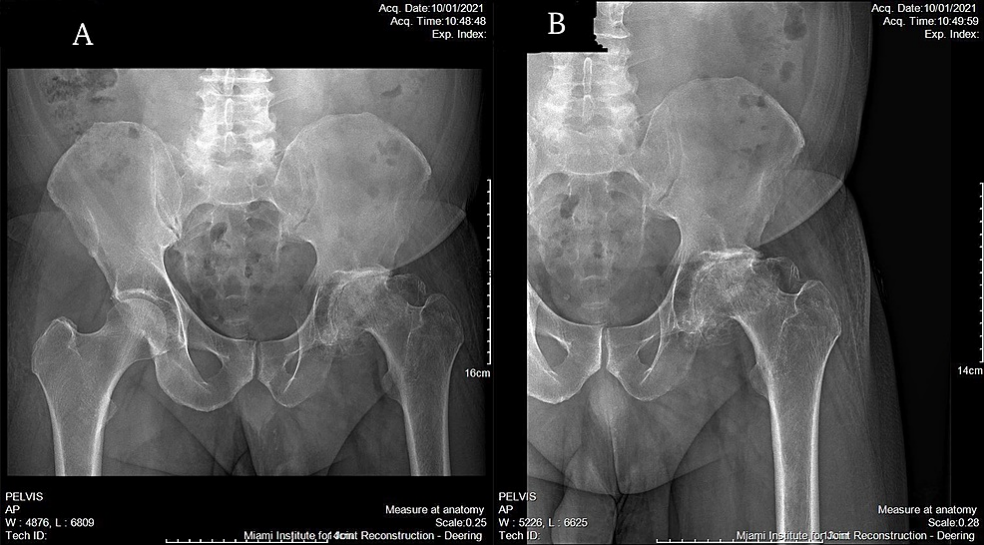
Preoperative anteroposterior radiographs of the (A) pelvis and (B) left hip.
